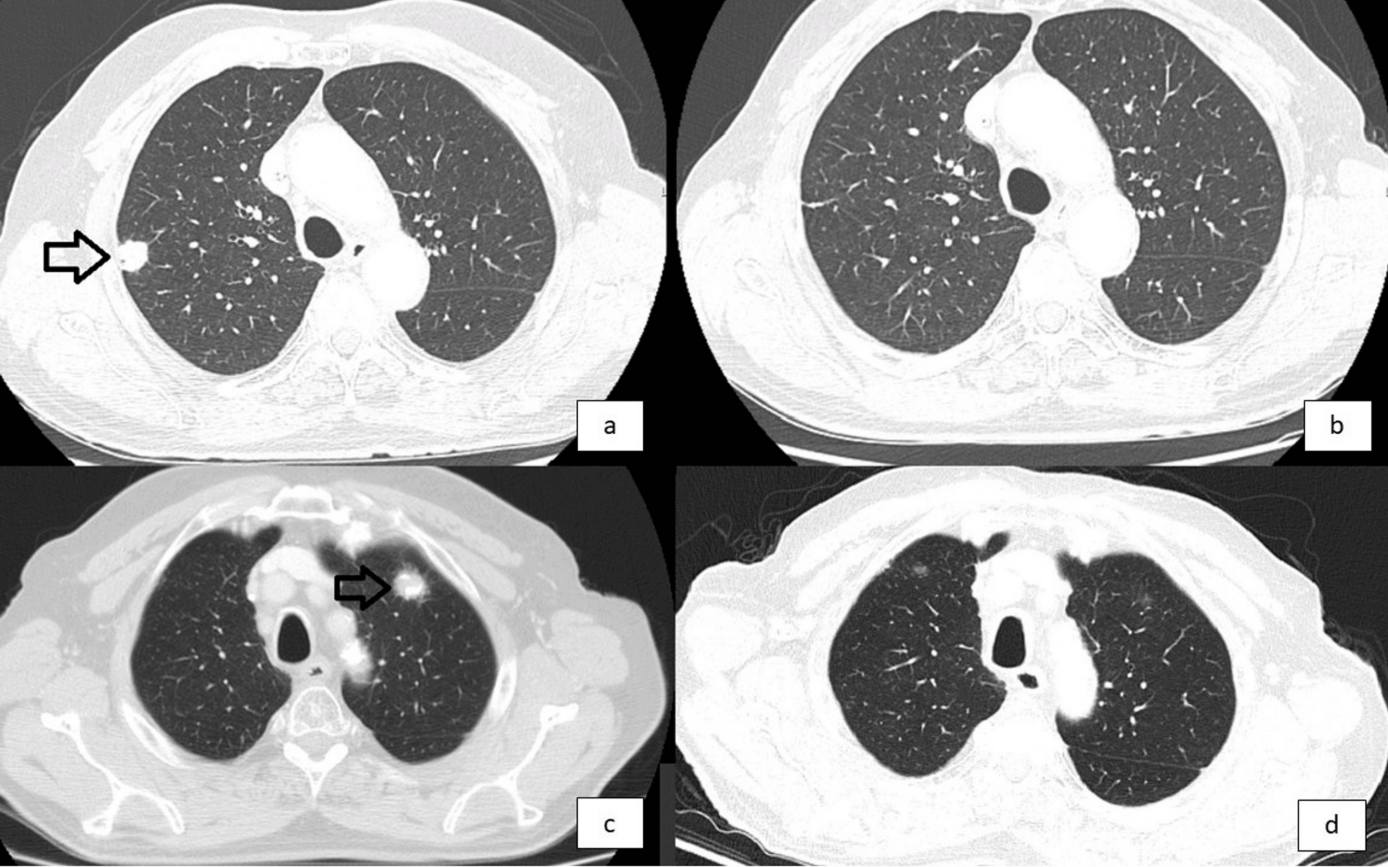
Comparison of pulmonary metastases before (May 2023) and after (September 2025) discontinuation of nivolumab therapy Panels A and C: Axial chest CT images demonstrating right upper lobe pulmonary metastases (arrows) before initiation of nivolumab. Panels B and D: Corresponding follow-up images showing a complete radiologic resolution of the metastatic lesions twenty-one months after suspension of nivolumab.
